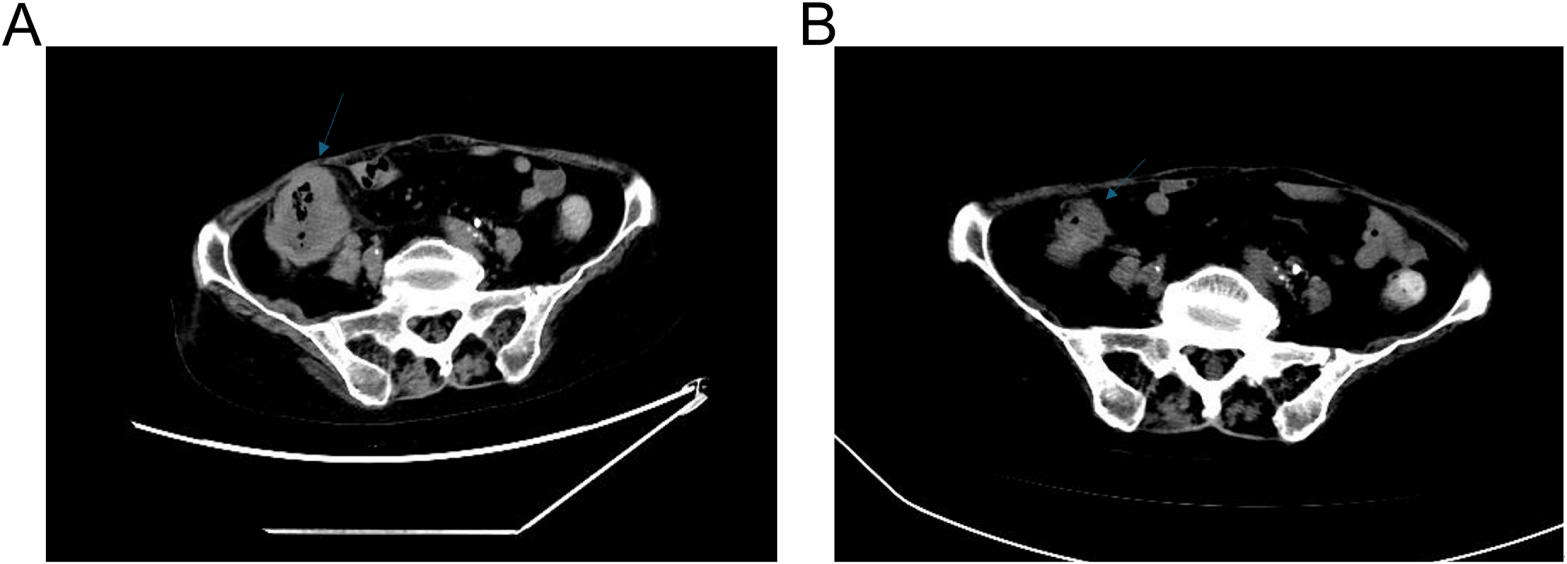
CT of the patient. (A) Abdominal CT scan prior to the treatment of tislelizumab. (B) Abdominal CT scan after the treatment of tislelizumab.
